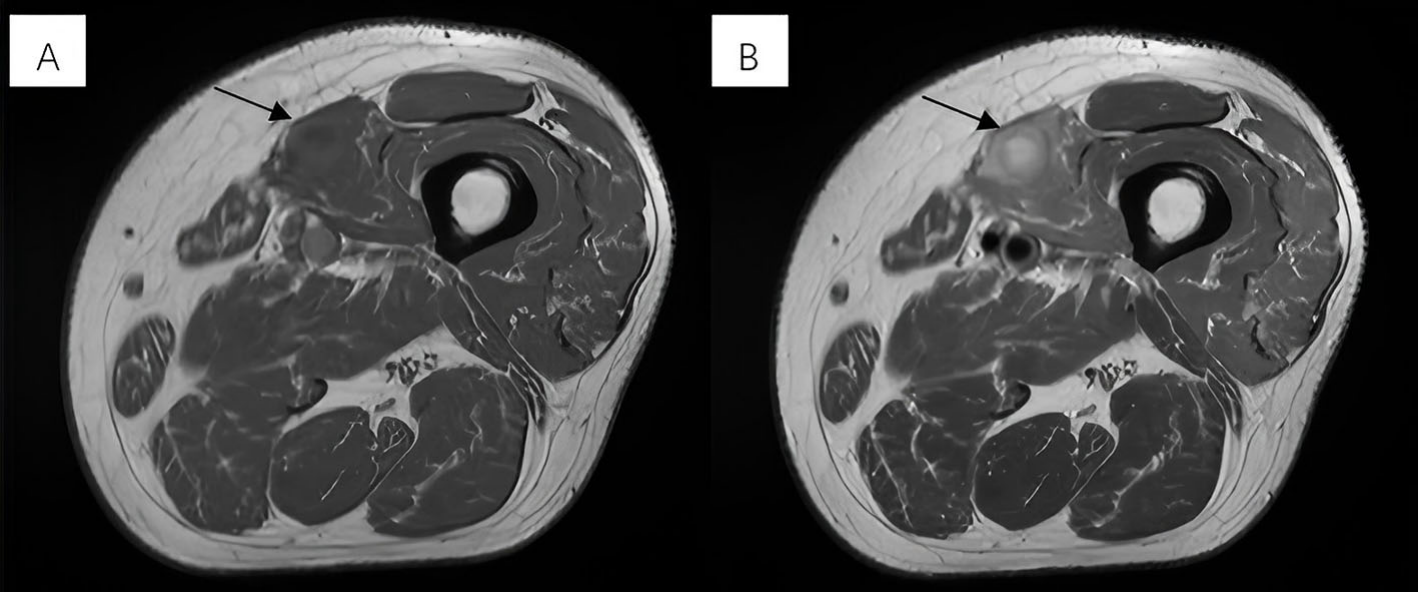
(A) Exhibiting iso-intensity on T1-weighted images. (B) Mixed hyperintensity on T2-weighted images.
